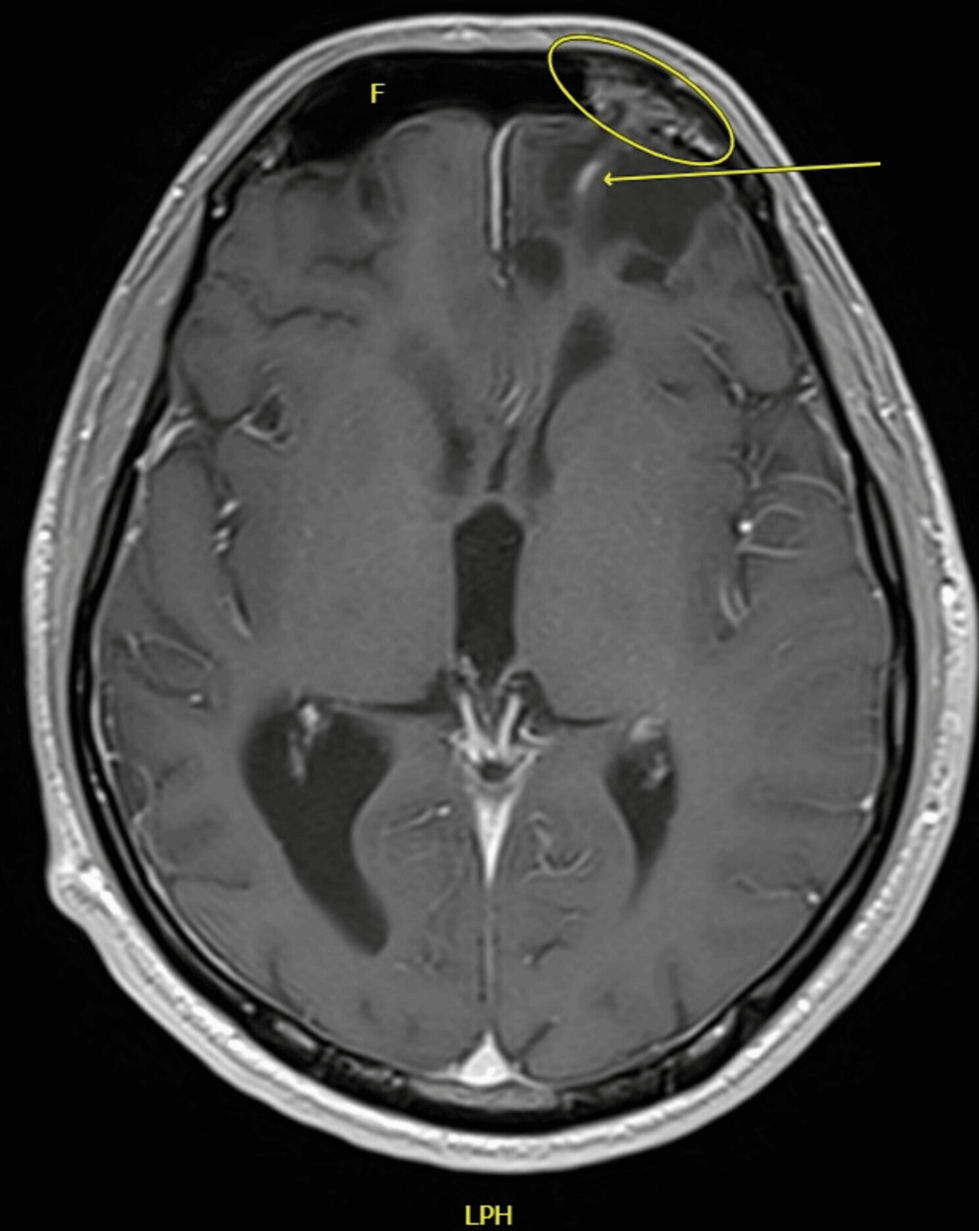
MRI scan’s T1 axial view with contrast showing residual linear peripheral enhancement (arrow) seen at the site of the previously noted left frontal ring-enhancing space-occupying lesion. Encephalocele (eclipse) is still seen in the left frontal sinus (F).
